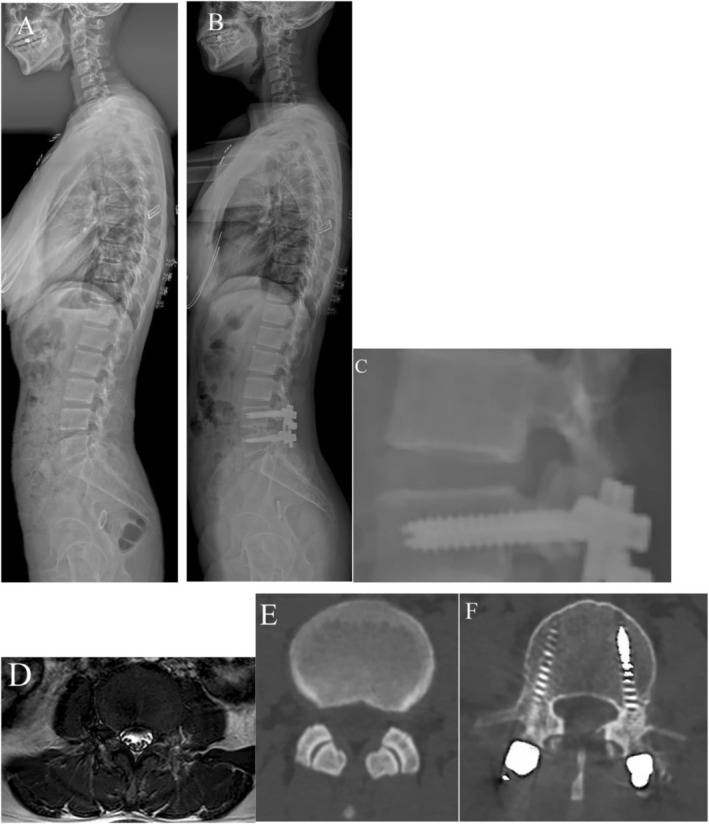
(A) A 48‐year‐old female patient diagnosed with L4/5 lumbar spinal stenosis. (B, C) Lateral radiograph at 3‐year follow‐up showing no radiographic evidence of degeneration at the adjacent L3/4 level. (D) Preoperative axial and sagittal MRI at L3/4 demonstrating well‐preserved paraspinal muscle morphology. (E, F) Preoperative CT shows no facet joint degeneration at L3/4, while postoperative CT reveals no facet joint violation at the same level.
